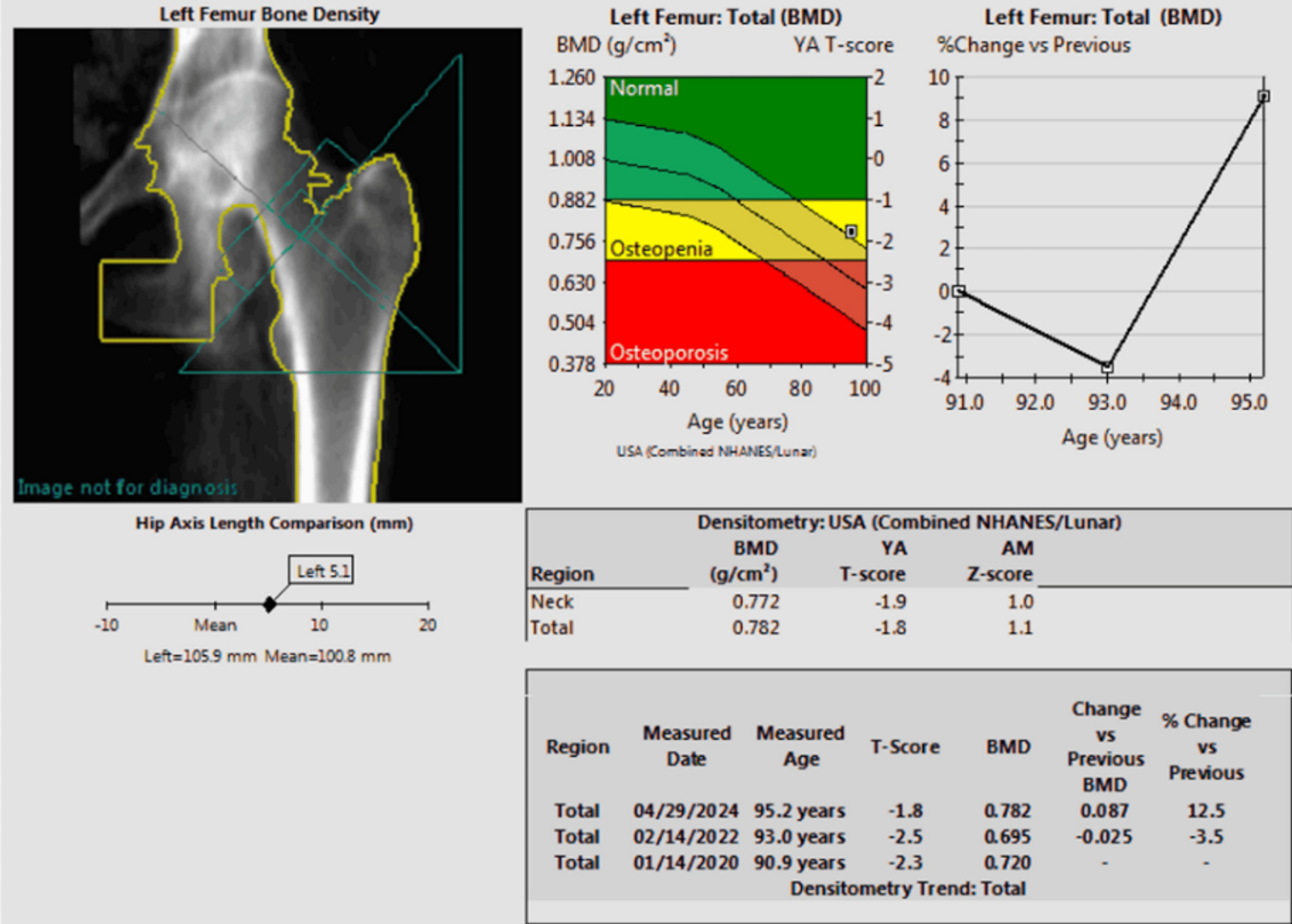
Posttreatment DEXA DEXA: Dual-energy X-ray absorptiometry; BMD: bone mineral density DEXA scan images obtained in 2024. Left femoral neck image reveals a BMD of 0.772 and a T-score of -1.9
